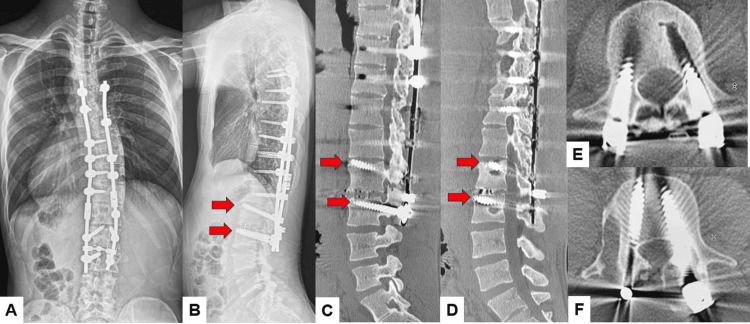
Postoperative images after revision surgery A: Postoperative posteroanterior whole spine radiogram, B: Postoperative lateral whole spine radiogram, C: Right parasagittal lumbar reconstruction CT, D: Left parasagittal lumbar reconstruction CT, and E and F: Axial CT at L3. Red arrows indicate transdiscal screws.
